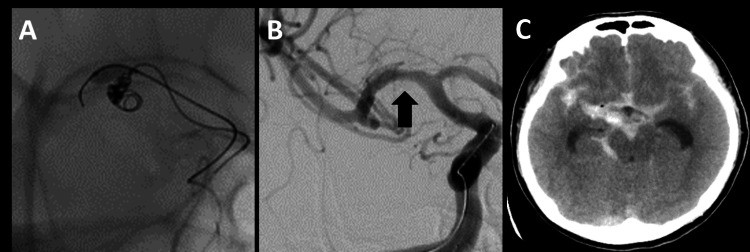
Endovascular intraoperative DSA and postoperative CT findings (A) Balloon-assisted coil embolization was performed. (B) DSA shows that the aneurysm has completely disappeared during the procedure (black arrow). (C) Postoperative CT showed no increased bleeding CT: computed tomography; DSA: digital subtraction angiography
